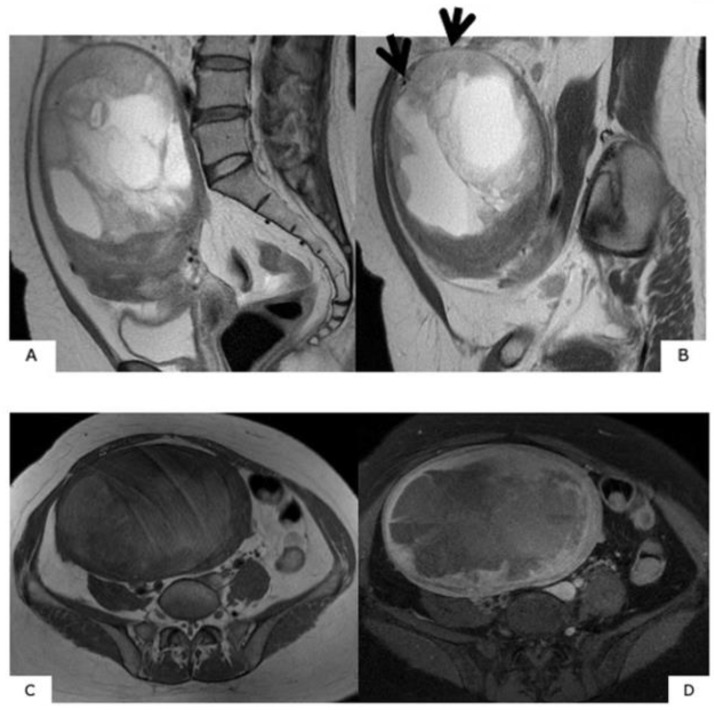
Magnetic resonance images of leiomyosarcoma (age, 49 years). (A,B): T2-weighted images sagittal sections, (C): T1-weighted axial section, (D): contrast T1 fat-suppression axial section. In the myometrium, heterogeneous high signals are presented with T2-weighted images, and with T1-weighted images, there is confirmation of mass showing faint high signals considered to be hemorrhage. In the contrast T1 fat-suppression image (D), a poorly contrasted area considered to be necrosis is found, while in (B), the fundus uteri side shows extremely thin myometrium, together with finding a portion considered to be extraserosal exposure, with ill-defined tumor borders (arrow).
